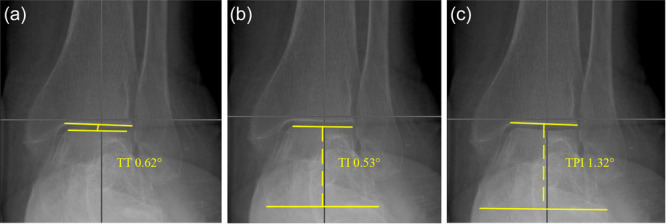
Ankle measurements on standing radiographs: (a) talar tilt (TT), (b) talar inclination angle (TI), and (c) tibial plafond inclination angle (TPI).
